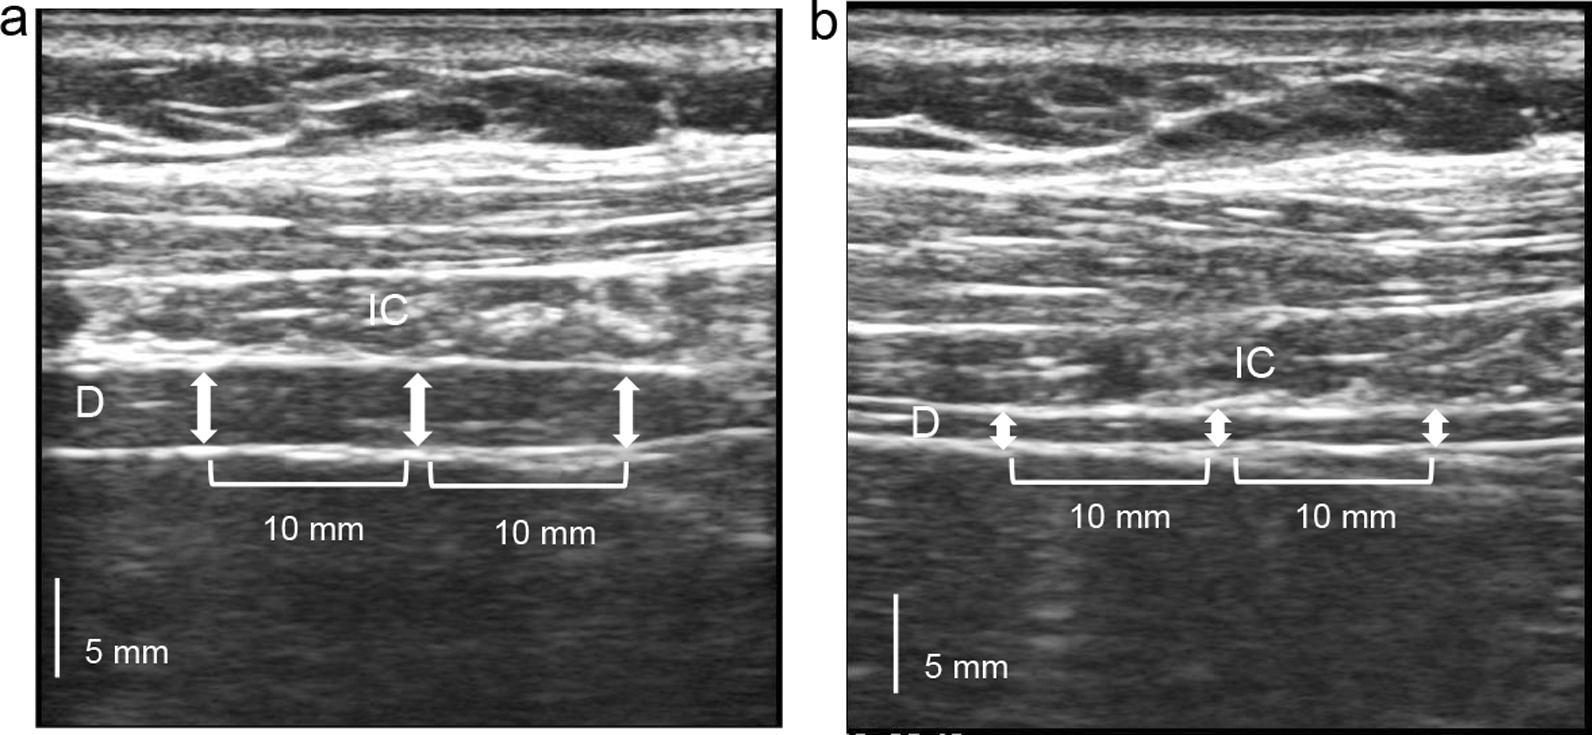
Representative ultrasound of the diaphragm. Diaphragm at total lung capacity (a) and functional residual capacity (b). The diaphragm (D, white bidirectional arrows) is the 3-layered structure situated deep to the intercostal (IC) muscles spanning two ribs; it is identified as the last set of parallel lines, with the pleural and peritoneal membranes overlying the less echogenic muscle. We calculated a mean of three points using the IMT software: the center of the diaphragm thickness, and two surrounding points on either side (each 10 mm from the center of the diaphragm thickness)
